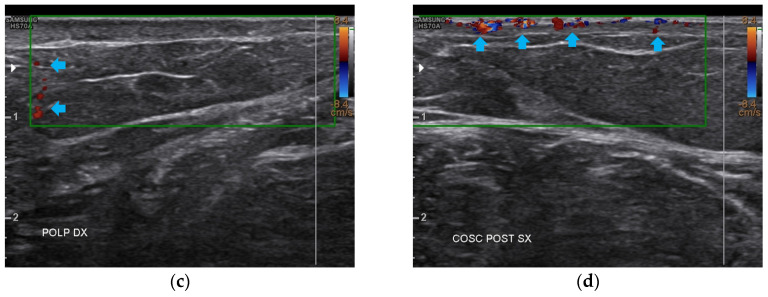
Doppler ultrasound of the four areas analyzed. (a) Right forearm; (b) Left popliteal fossa; (c) Right calf; (d) Left posterior thigh. All the areas present a clear revascularization (blue arrows) extending to the deeper layers, particularly on the popliteal fossae and calves.
